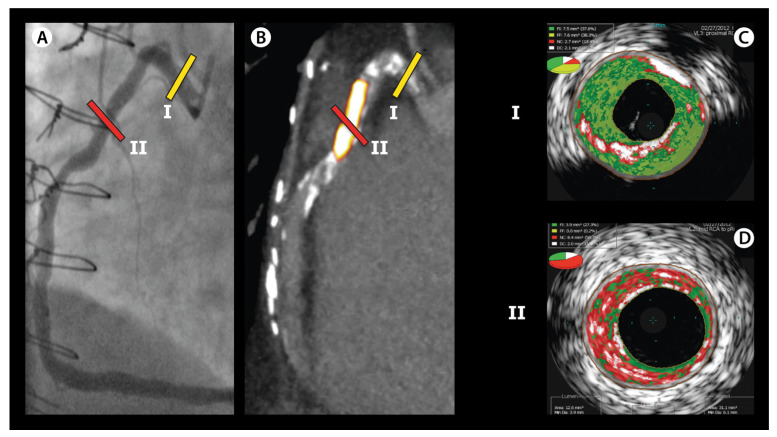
Vulnerable plaque on ^18^F-NaF PET imaging. In (A), coronary angiography demonstrates two non-obstructive lesions in the proximal and mid-right coronary artery in a patient with stable angina. (B) demonstrates the corresponding ^18^F-NaF PET-CT image, which indicates no uptake of ^18^F-NaF in lesion I, but an increased uptake in lesion II. (C) and (D) demonstrate the corresponding radiofrequency IVUS images. Lesion I (C) appears to be a lesion consisting of fibrous tissue (green) with confluent calcium (white) with acoustic shadowing. However, the ^18^F-NaF positive lesion II (D) appears to consist of a large necrotic core (red), with microcalcifications (white), suggestive for a vulnerable plaque. Image adapted from Joshi NV, et al. [139] CC-BY [4.0].
